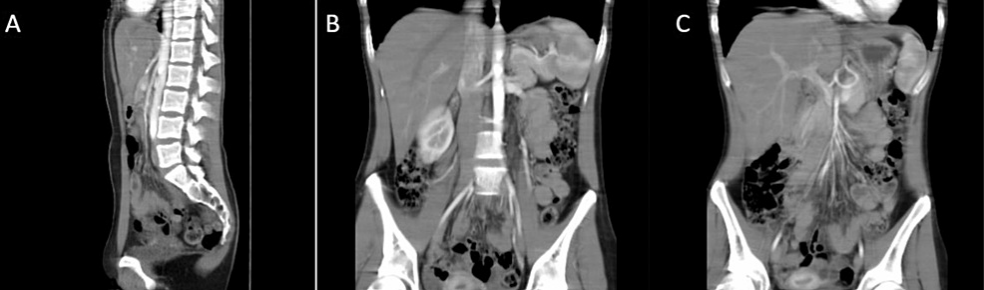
CT angiography of the abdomen and pelvis in multiple views. Lateral (A) and Coronal (B) views reveal an acute angle of the superior mesenteric artery together with compression of the second portion of the duodenum (C) by the superior mesenteric artery. A. CT angiography lateral view of the abdomen and pelvis revealing an acute angle of the superior mesenteric artery, as well as patent celiac axis, superior mesenteric artery, and inferior mesenteric artery B. CT angiography coronal view of the abdomen and pelvis revealing an acute angle of the superior mesenteric artery. C. CT angiography of the abdomen and pelvis showing compression of the second portion of the duodenum by the superior mesenteric artery.
